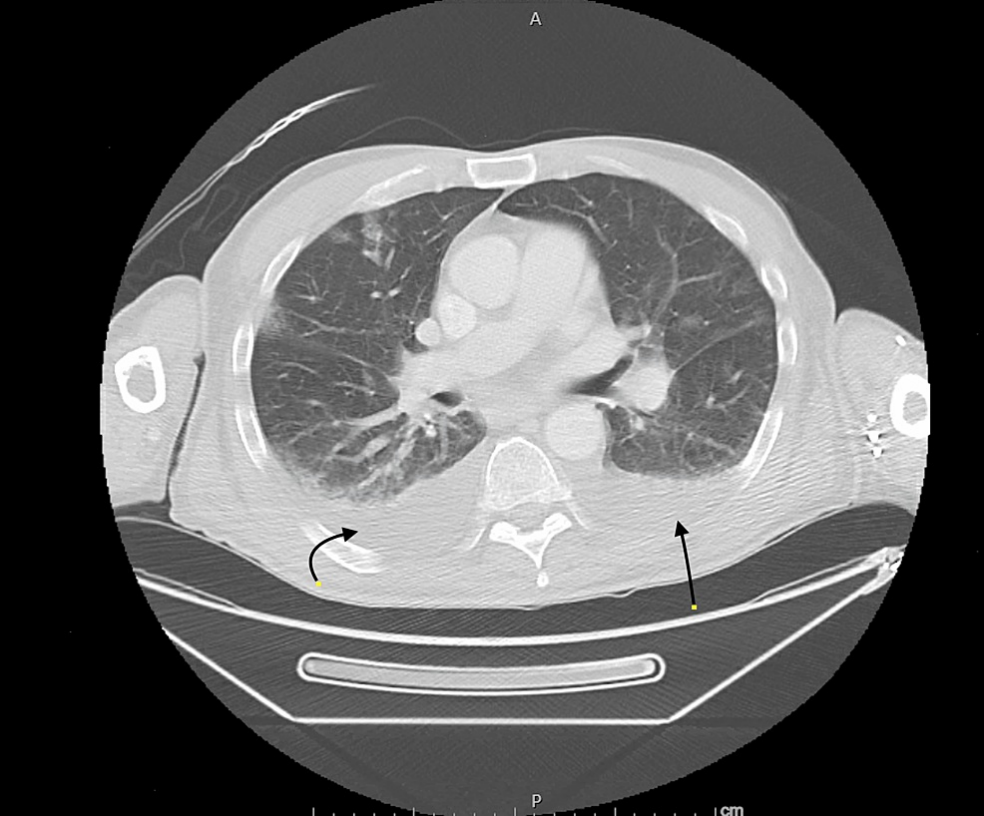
CT of the chest: axial view Bilateral pleural effusions indicated with black arrows (chronic) CT: computed tomography
